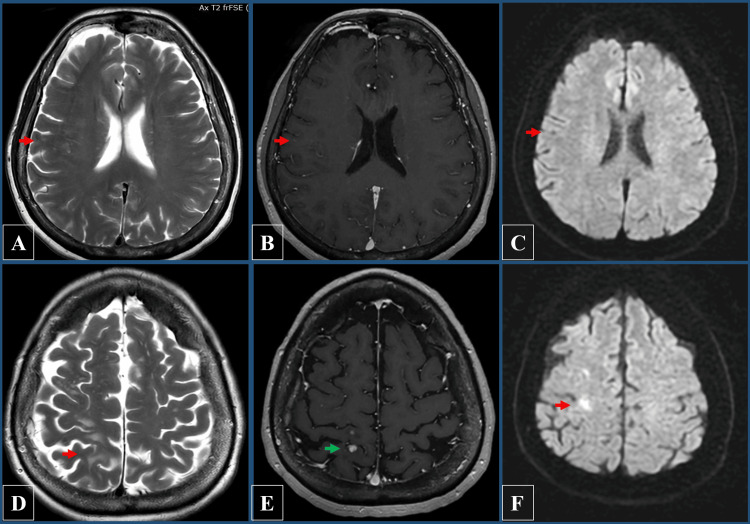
Magnetic resonance imaging (MRI) demonstrating axial images at one-year follow-up. (A, D) T2 fluid-attenuated inversion recovery (FLAIR) images showing a reduction in peri-lesional edema (red arrows). (B, F) T1-weighted post-contrast images showing a decrease in the number of nodular enhancing lesions (red arrow), while new lesions emerged with the most prominent being 4 mm in diameter (green arrow). (C, F) Diffusion-weighted imaging (DWI) showing the lesions with restricted diffusion (red arrows).
